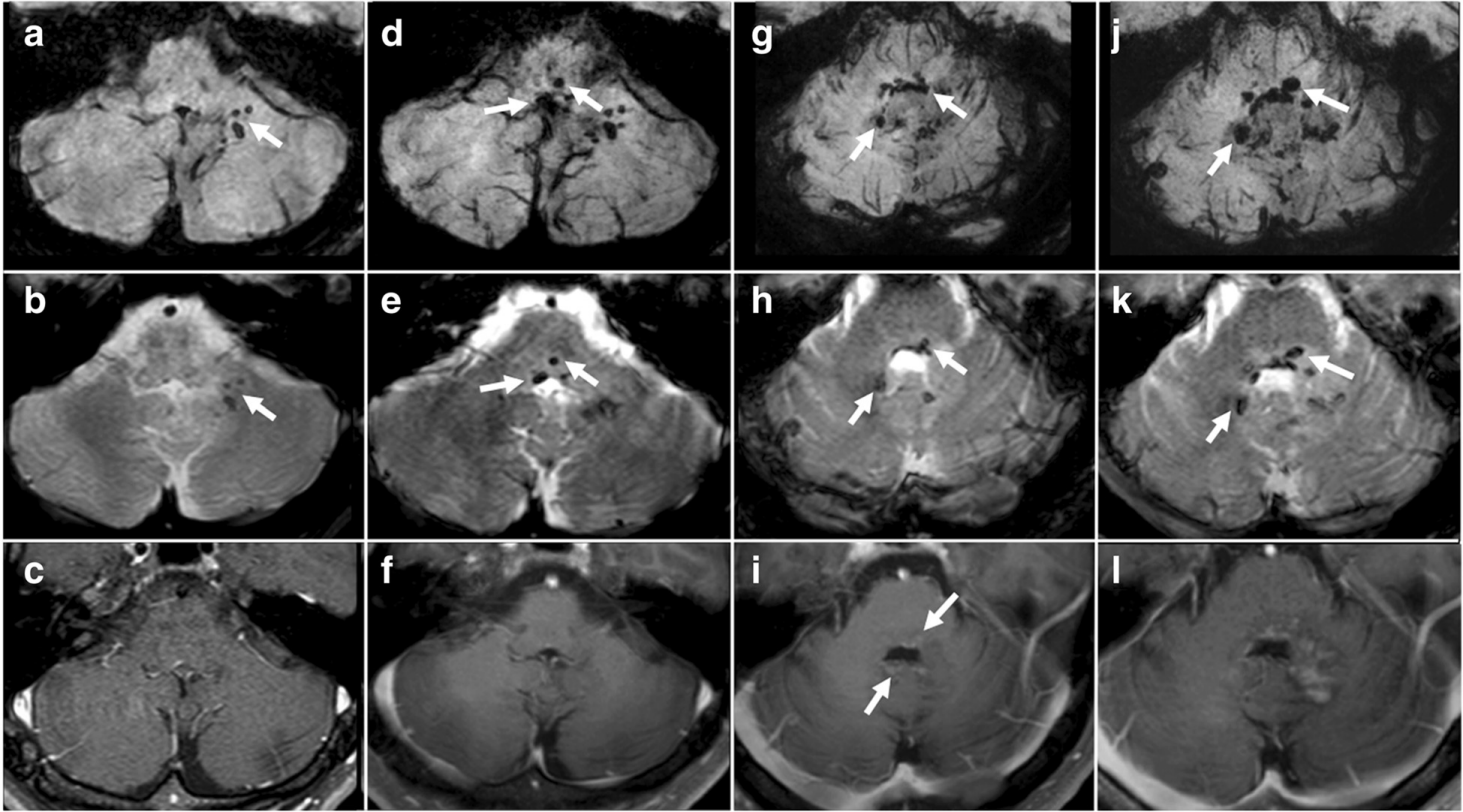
a SWI on the first admission revealed low-signal intensity spotty lesions in the left cerebellar peduncle (arrow). b T2*WI on the first admission. Findings similar to those in Fig. 1a are present (arrow). c Contrast-enhanced MRI on the first admission showed no apparently enhanced lesions, including in the cerebellar peduncle. d SWI obtained three months after the first admission revealed de novo low-intensity spots in the medulla oblongata. e T2*WI obtained three months after the first admission. Findings are to those in Fig. 1d. f Contrast-enhanced MRI obtained three months after the first admission showed no apparently enhanced lesions. g SWI obtained one year after the first admission revealed spreading hypointensity- lesions adjacent to the fourth ventricle (arrows). h T2*WI obtained one year after the first admission. Findings similar to those in Fig. 1g are present. i Contrast-enhanced MRI obtained one year after the first admission showed a slightly enhanced lesion around the fourth ventricle. j SWI on the second admission revealed expanding lesions of the pons and right cerebellar peduncle (arrows). k T2*WI on the second admission revealed findings similar to those in Fig. 1k. l Contract-enhanced MRI on the second admission showed an expanded enhanced lesion around the fourth ventricle
